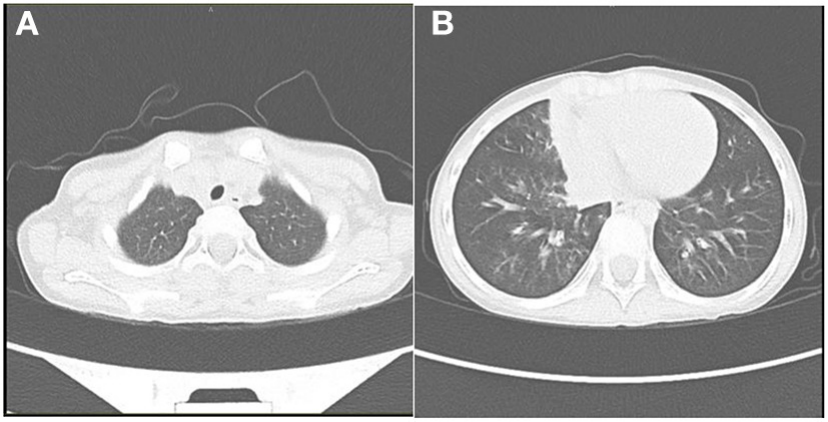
Patient's chest CT showed a few inflammatory lesions in the right upper lobe (A), the middle lobe, and the lower lobe of both lungs and the lower lobe of both lungs (B) on the first day of admission.
